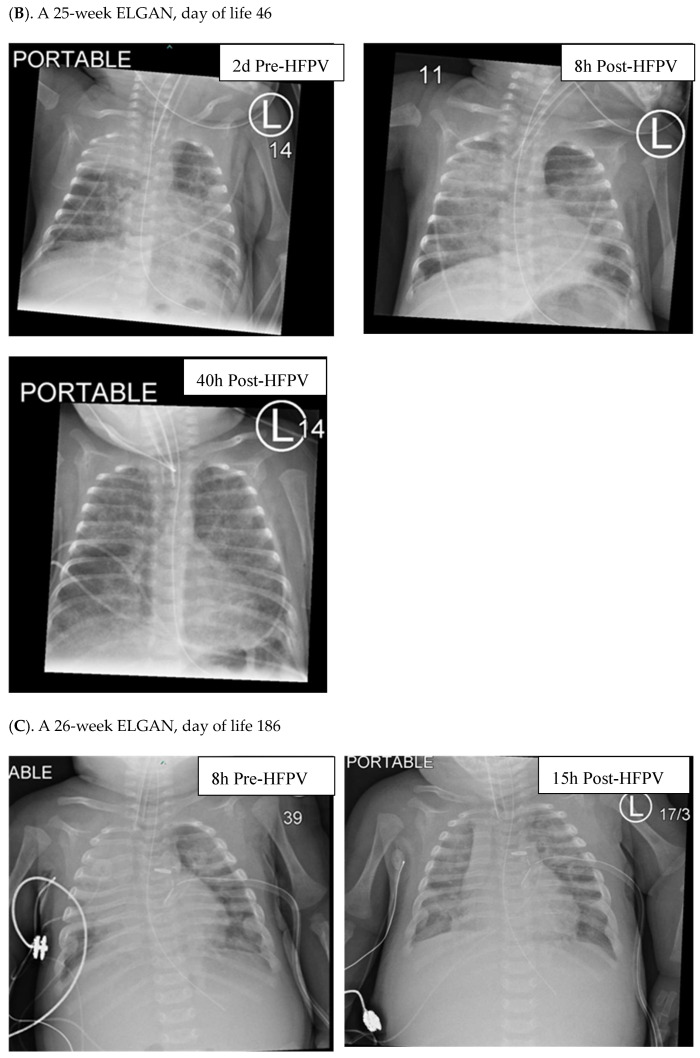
Radiographs of ELGANs placed on HFPV. Patient (A) is a 25-week gestation infant who was found to have worsening diffuse atelectasis with almost complete opacification of lung fields on a chest X-ray taken 8 h prior to placement on HFPV on DOL 46. Within 6 h after being placed on HFPV, the infant had a dramatic improvement in his clinical status with decreased FiO_2_ requirements and improved findings on CXR as (shown on the right). Patient (B) is a 25-week gestation infant changed to HFPV on DOL 46. CXRs performed 2 days prior, and 8 and 40 h post-placement on HFPV revealed a more gradual clearing of opacified lung fields. Patient (C) is a 26-week gestation infant with shifting areas of atelectasis, who was changed to HFPV on d186 (left). CXRs 8 h prior to placement on HFPV and 15 h following HFPV, respectively, revealed shifting and then improved aeration.
